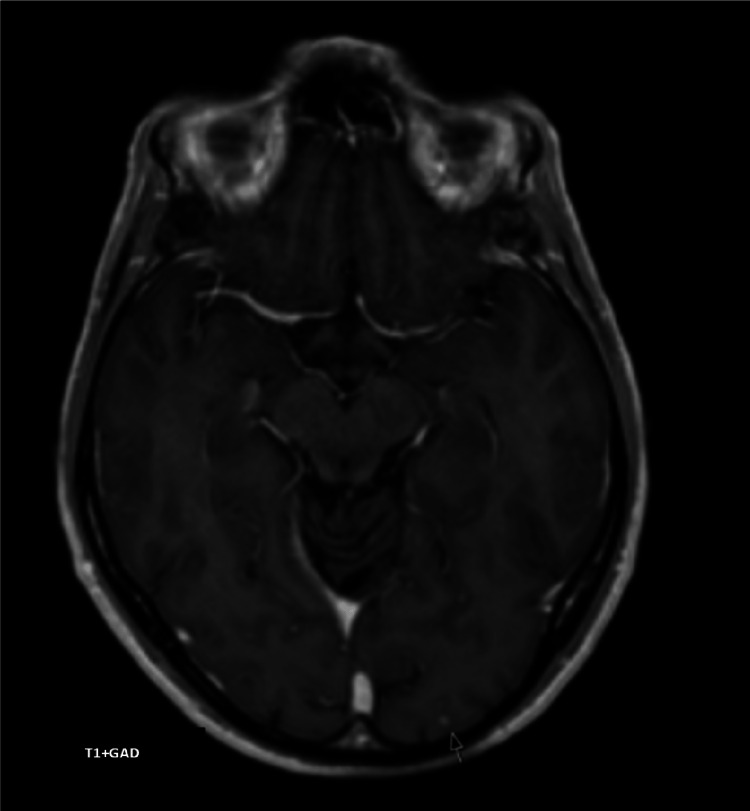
Brain magnetic resonance imaging Lesion with nodular contrast enhancement in the left occipital location (arrow), with a marked reduction in its dimensions compared to the previous image. No halo of vasogenic edema.
